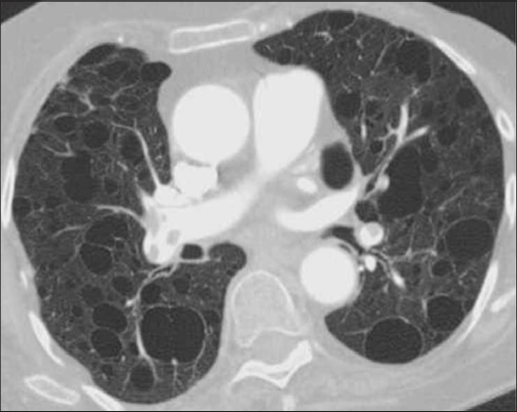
LAM.* An 84-year-old female, previously operated on for an angiomyolipoma, was admitted with dyspnea. CT showed multiple thin-walled cysts with varying sizes.
